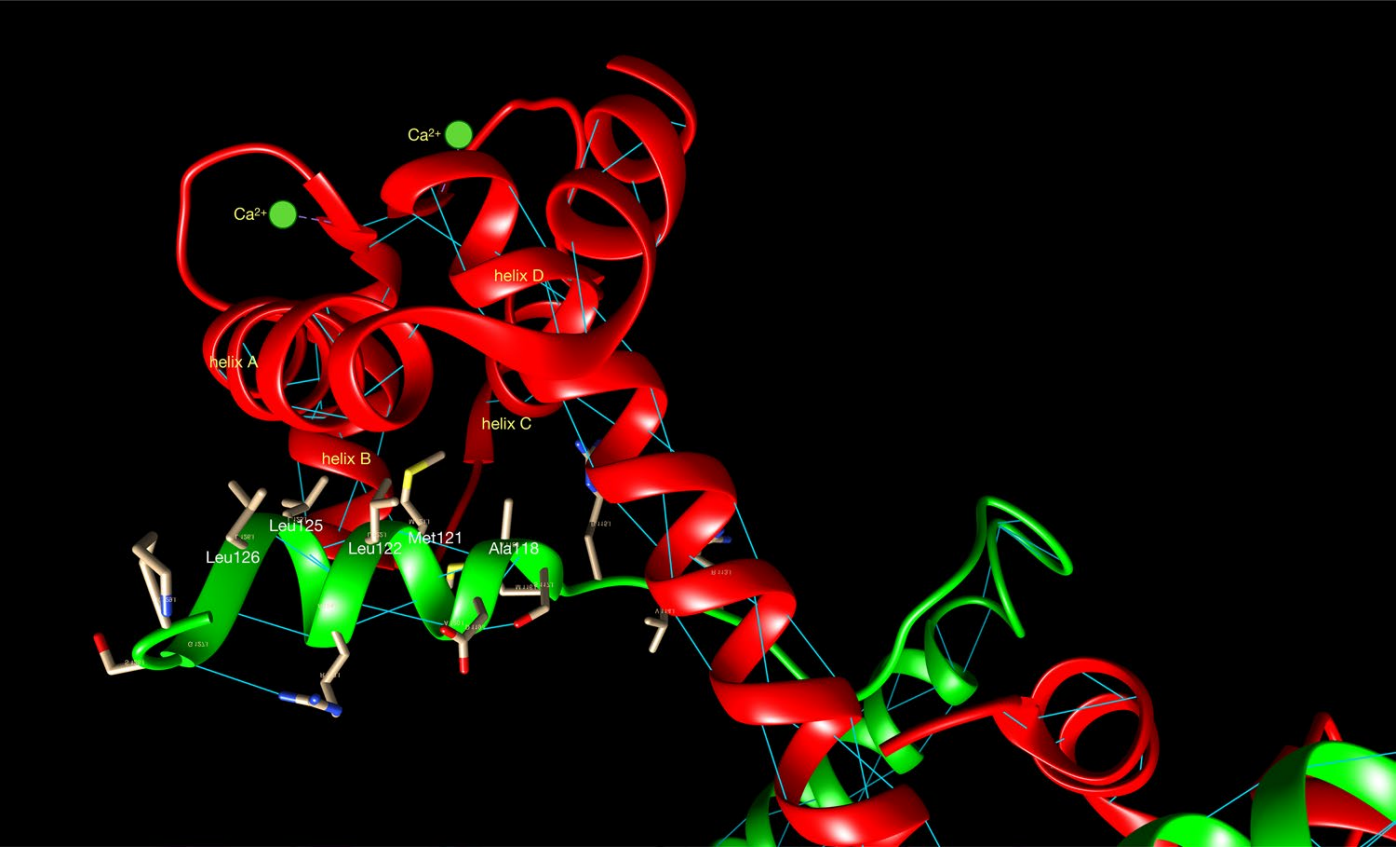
The binding of the skeletal muscle TnI switch peptide, helix3 (green), to the hydrophobic patch of skTnC- Ca^2+^ (red). The switch peptide is an amphipathic helix with hydrophobic residue on the face interacting with TnC (Ala118, Met121, Leu122, Leu125, Leu126). Rendered from Vinogradova’s X-ray structure 1ytz with segments of TnI C-terminal to Helix 3 and TnT are removed for clarity. (Color figure online)
